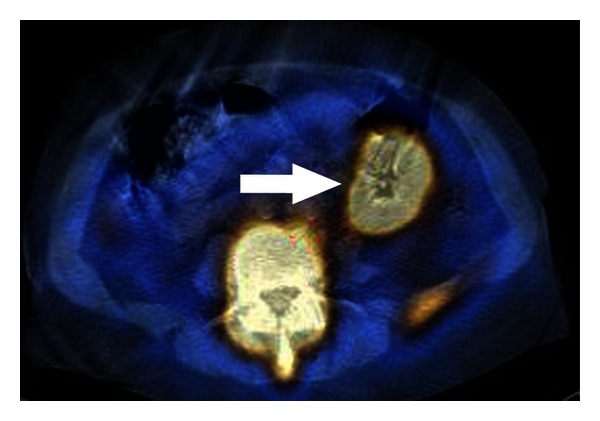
The same patient, abdomen SPECT/CT, transversal slice. Granulocyte uptake in the ileum—m. Crohn (*arrow head*). Normal uptake of the radiotracer in bone marrow.
